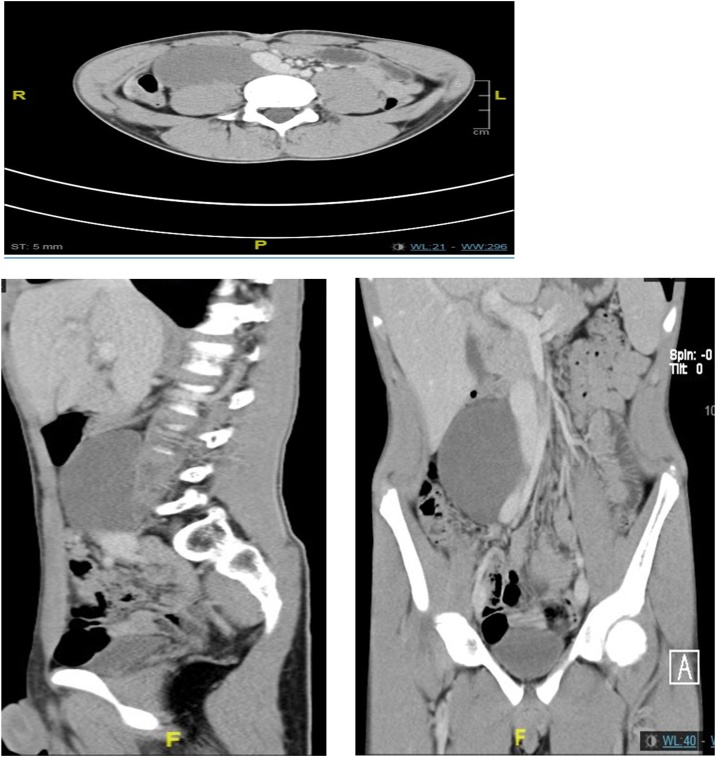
CT abdomen showing a cystic lesion at lower right retroperitoneal space, near the inferior vena cava and right iliac vessels.
